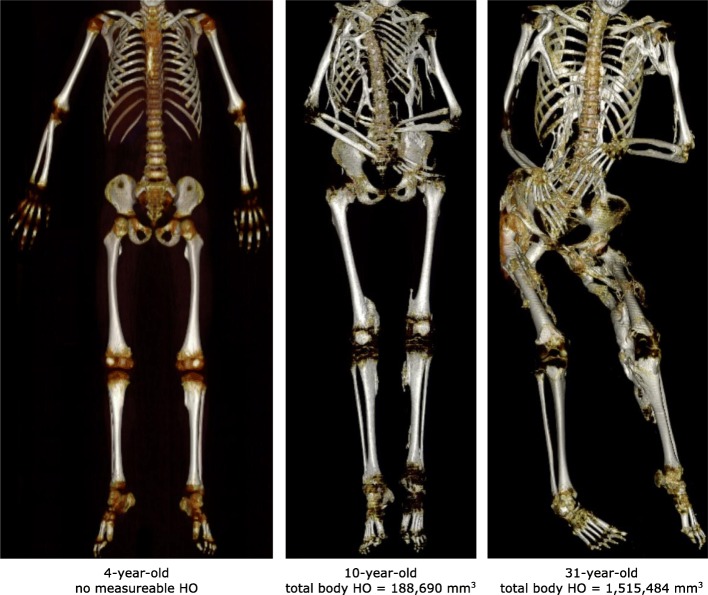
Whole Body Computed Tomography Images from Representative Subjects
